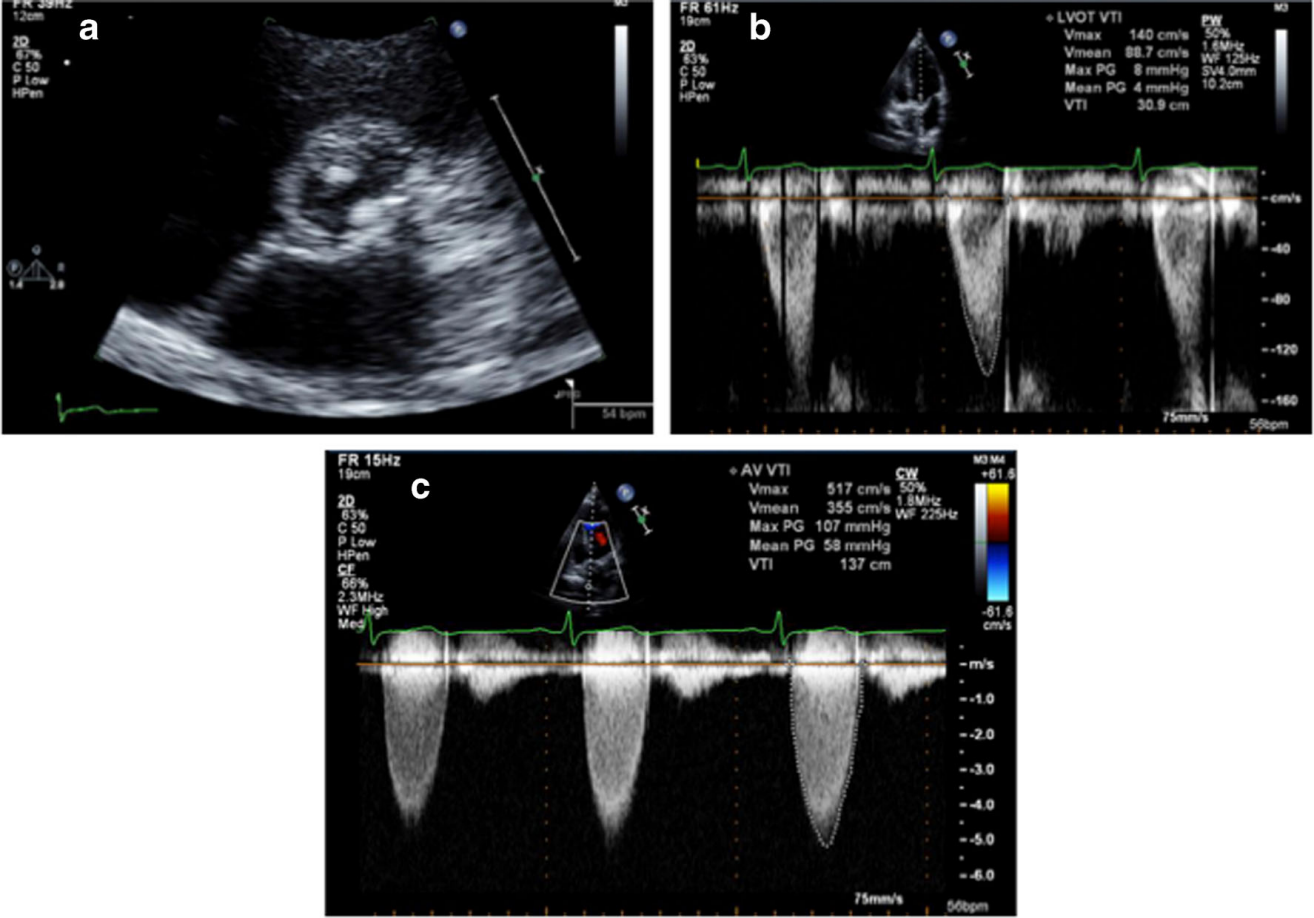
A 31-year-old pregnant woman with a history of St. Jude 21-mm bioprosthetic aortic valve replacement in 6 years ago (a, short-axis view) was found to have elevated gradients across her aortic valve on routine echocardiogram during her first trimester with an aortic valve area of 0.75 cm^2^ by continuity equation indicating severe aortic stenosis. Doppler velocity index—a ratio of PW Doppler subvalvular velocity (b) and the maximum CW Doppler velocity across aortic valve (c) was 0.25 indicating nonsevere aortic stenosis. Patient was followed closely throughout pregnancy and had normal vaginal delivery without any complication
